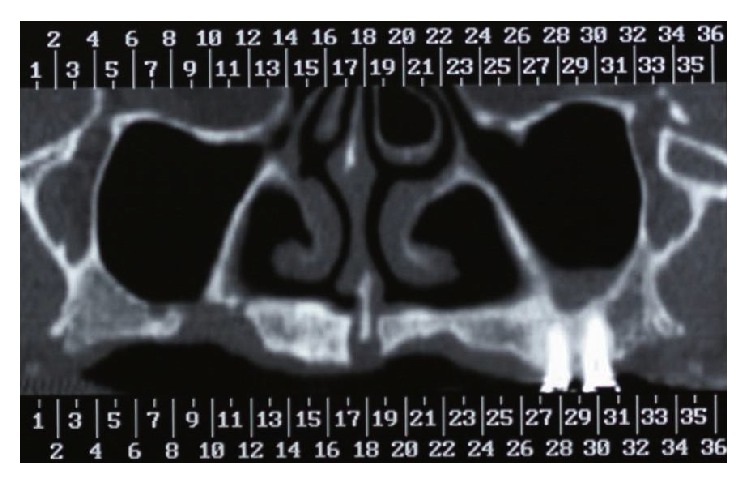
Computed tomography of maxilla. Distance between reconstructions: 3 mm.
